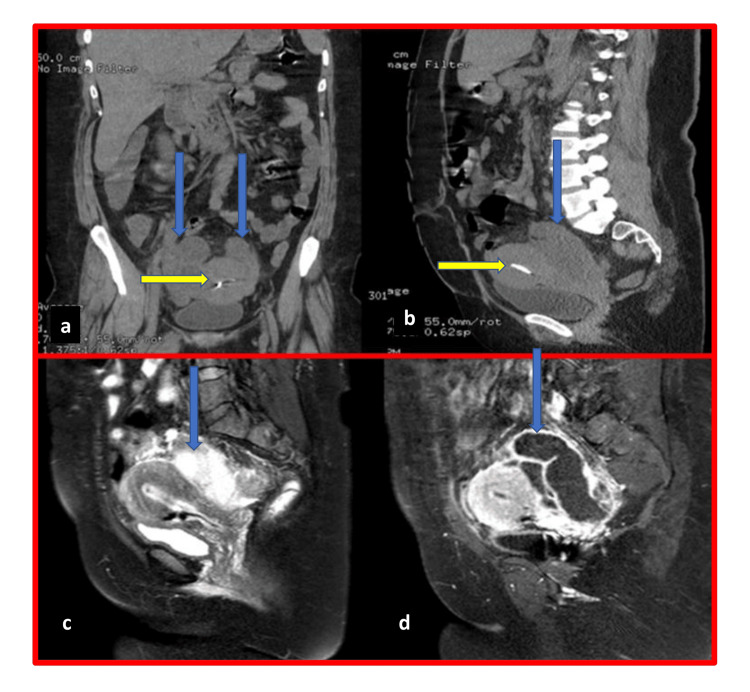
CT scan coronal sections showing IUCD, uterus, and overdistended tubes (a and b) Intrauterine contraceptive device (IUCD) inside the uterus (yellow arrow) and over distended tubes (blue arrows). (c and d) Over distended tubes (blue arrows).
